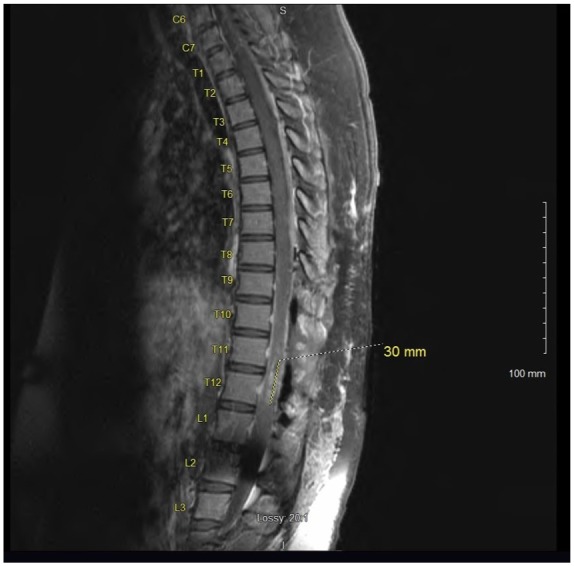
Postoperative MRI of the thoracic spine with contrast, postoperative day 1 of T9-T12 and L1 laminectomy with debulking of intramedullary spinal cord GBM. In this image, the residual tumor measures 30 mm in craniocaudal span.
